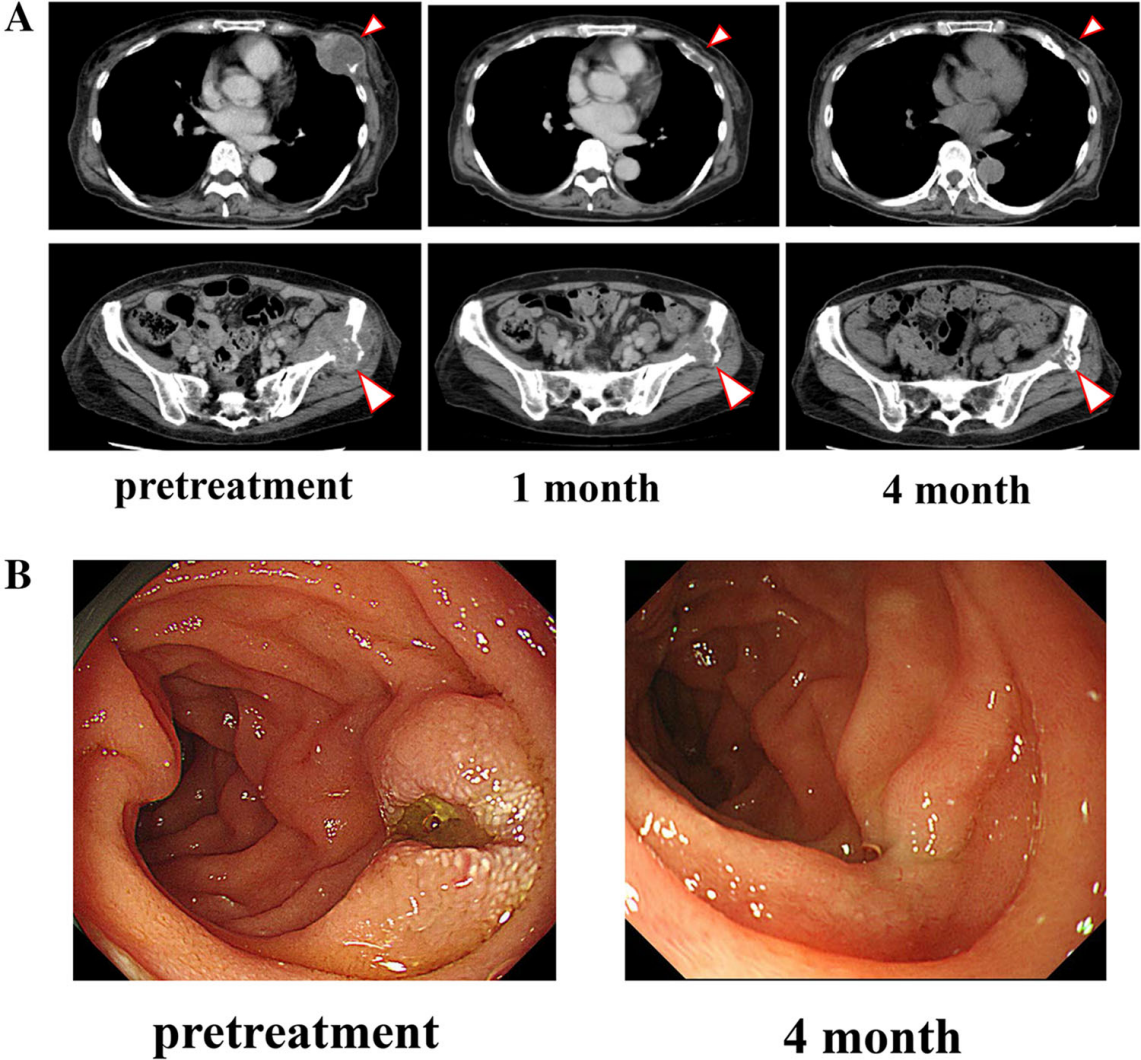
Demonstration of treatment response. a Computed tomographic (CT) scan with soft tissue windows of the bone metastasis. The rib and ileal lesions show acceptable reductions 1 month after the first treatment and nearly CR state was continued after 4 month. b The endoscopic image before and 4 month after the treatment
